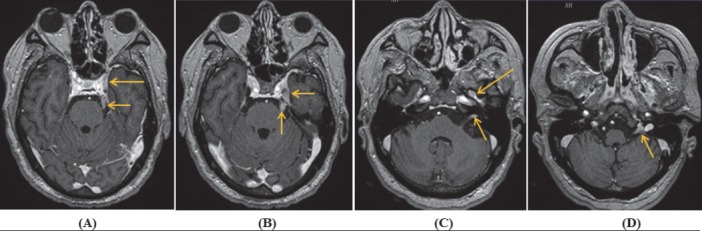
Axial contrast-enhanced T1W MRI showed enlargement and enhancement of the left cranial nerves: (A) complex of cranial nerves III, IV, VI, V1, V2 (long arrow); (A, B) cranial nerves V (short arrows); (C) cranial nerve V3 (long arrow) and complex of cranial nerves VII/VIII (short arrow); and (D) complex of cranial nerves IX, X, XI
